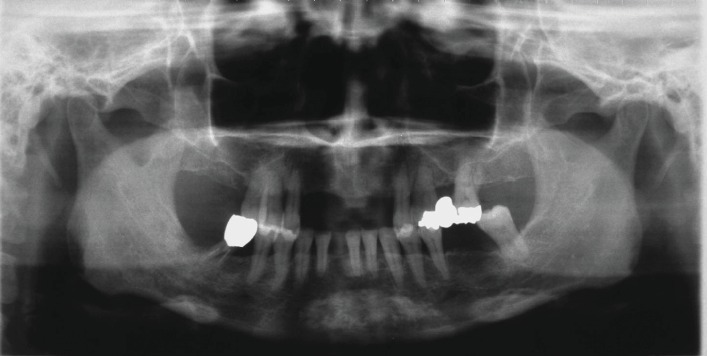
Reevaluation radiographs. Panoramic radiography revealed partially edentulous maxilla and mandible.
